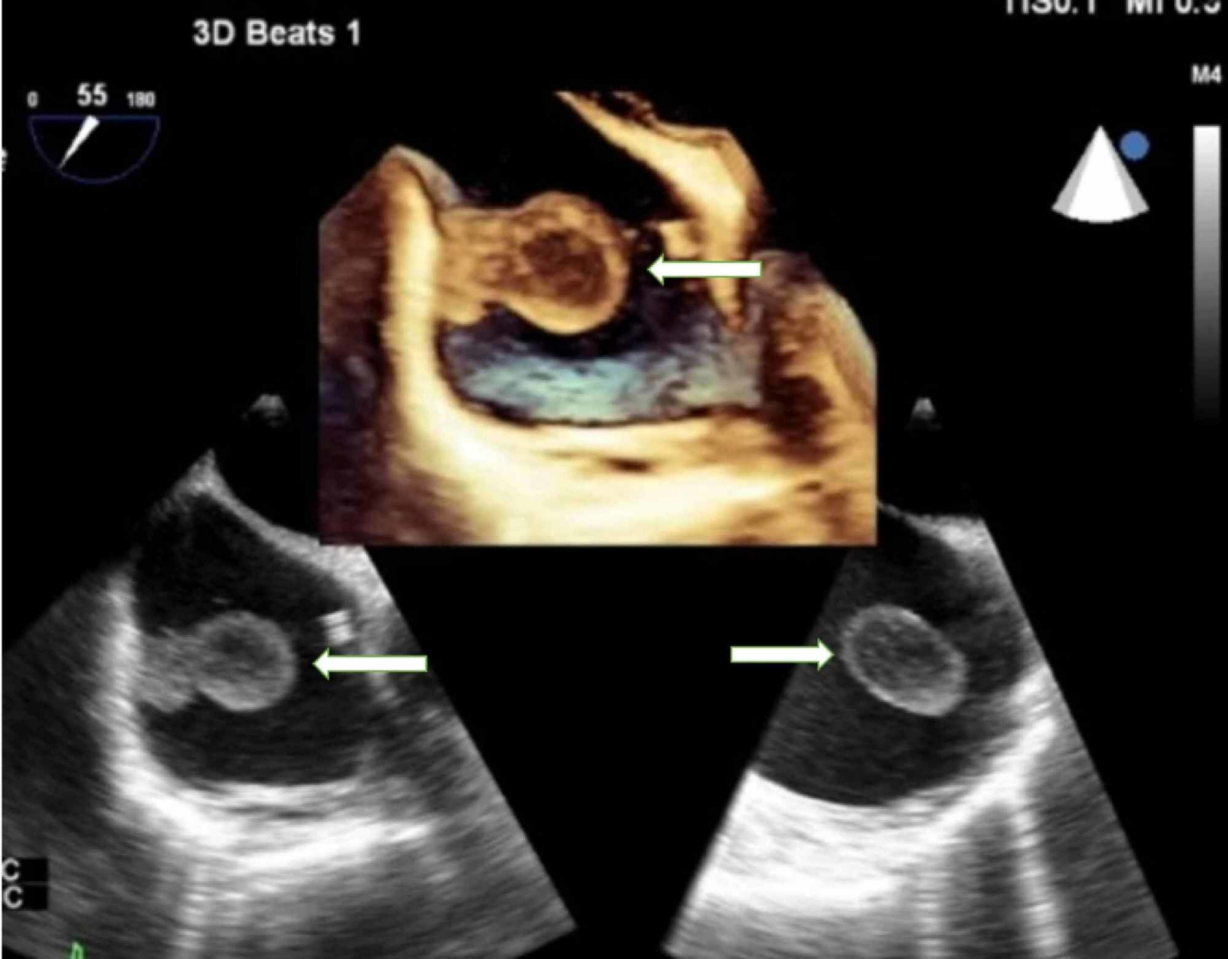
Two-dimensional transesophageal echocardiogram The image shows the mid-esophageal bicaval view of the atrium demonstrating RA thrombus arising from the right atrial free wall, measuring 1.9 x 3.6 cm (arrows). Inset shows three-dimensional TEE showing RA thrombi (arrow) TEE: transesophageal echocardiogram; RA: right atrial
